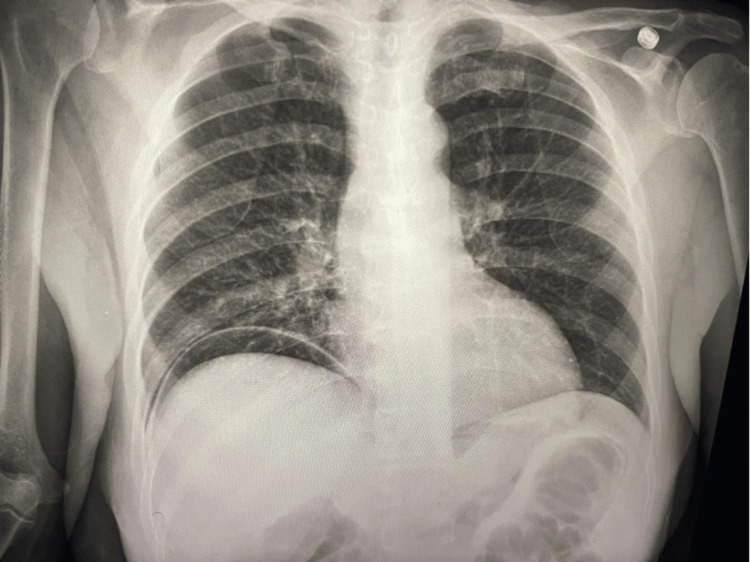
Upright chest radiograph demonstrating free subdiaphragmatic air consistent with pneumoperitoneum.
